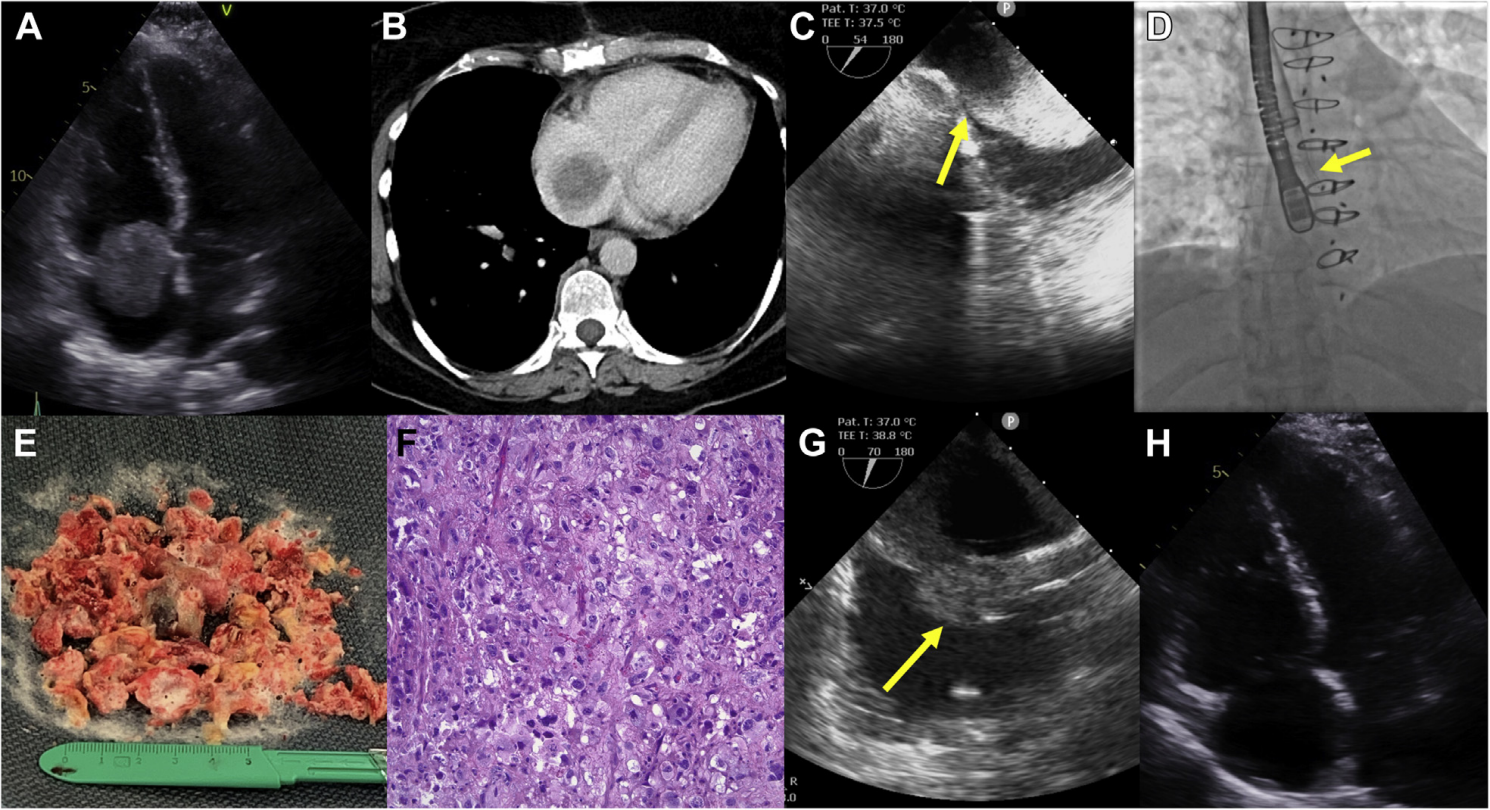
Transcatheter right atrial tumor debulking using the AngioVac device. (A) Apical 4-chamber view demonstrating a 4.7-cm right atrial mass protruding through the tricuspid valve. (B) Cross-sectional computed tomography demonstrating a large filling defect in the right atrium. (C) Modified view from the intraprocedural TEE showing the mass attached to the inferior vena cava and right atrial junction (yellow arrow). (D) Fluoroscopy showing the AngioVac device (yellow arrow) in the right atrium. (E) Gross image of the tissue retrieved. (F) High power magnification (200×) of tumor cells demonstrating marked nuclear atypia. (G) Modified TEE view postintervention showing the remaining stalk of the tumor (yellow arrow). (H) Postprocedure apical 4-chamber view demonstrating that the mass was no longer evident. TEE, transesophageal echocardiogram.
